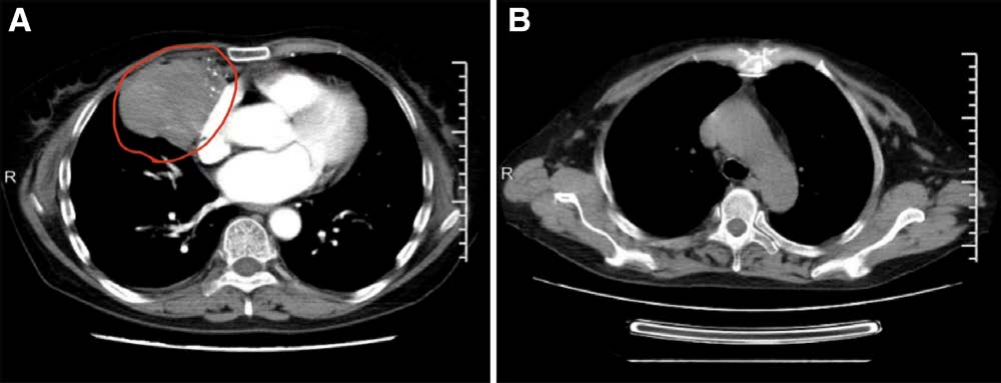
Computed tomography (CT) images of the patient. (A) CT showing an anterior mediastinal mass (7.6 × 6.3 cm). (B) Anterior mediastinal mass disappeared after thymectomy.
